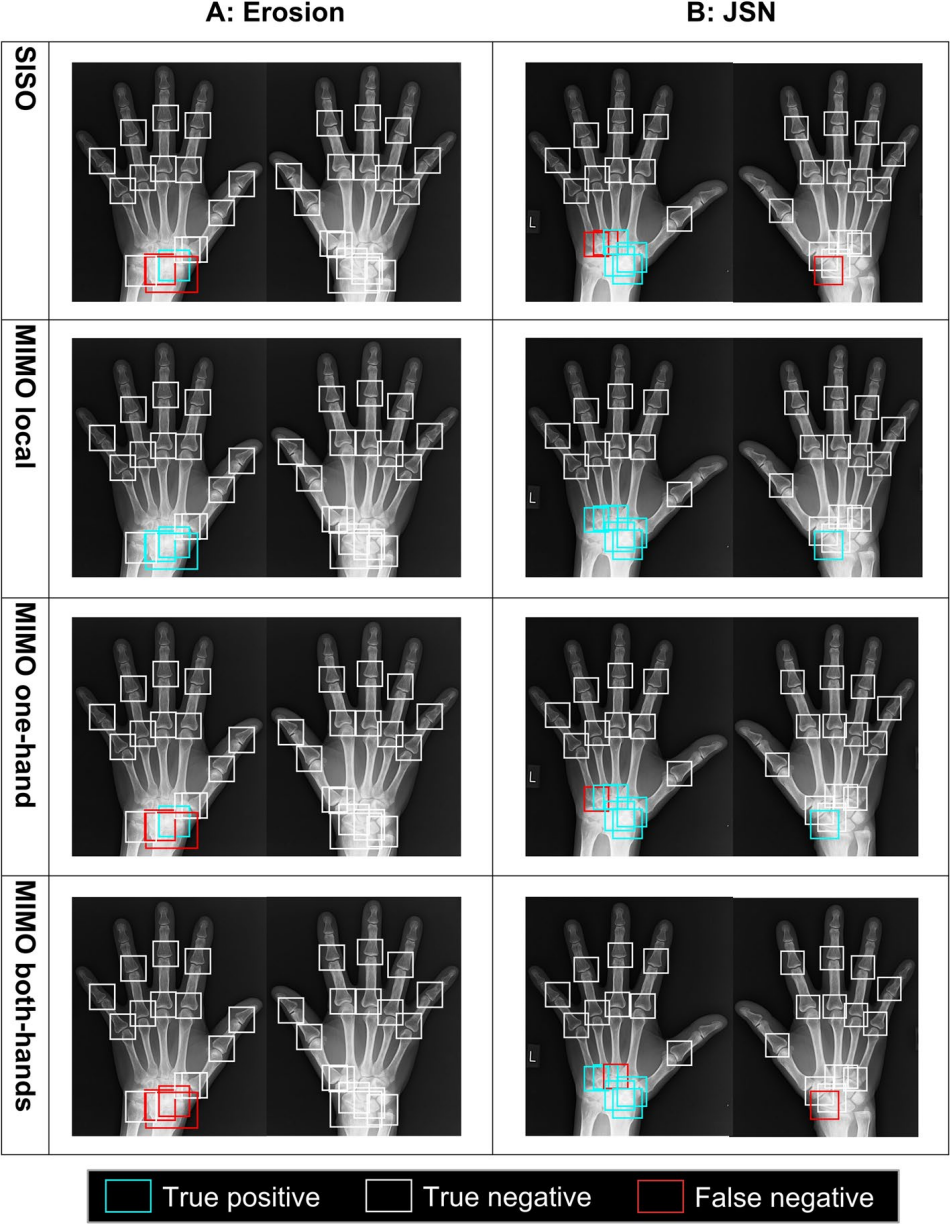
Visualization results of prediction by each classification model of target joints in X-ray images. Each bounding box represents the detected target joints. The color of the bounding box indicates how the classification results compare to ground-truth (GT). Blue indicates GT is non-intact, and prediction is non-intact (true positive). White indicates GT is intact, and prediction is intact (true negative). Red indicates GT is non-intact, and prediction is intact (false negative). A The results for erosion and B for JSN. GT, ground-truth; JSN, joint space narrowing; SISO, single-input single-output; MIMO, multiple-input multiple-output
